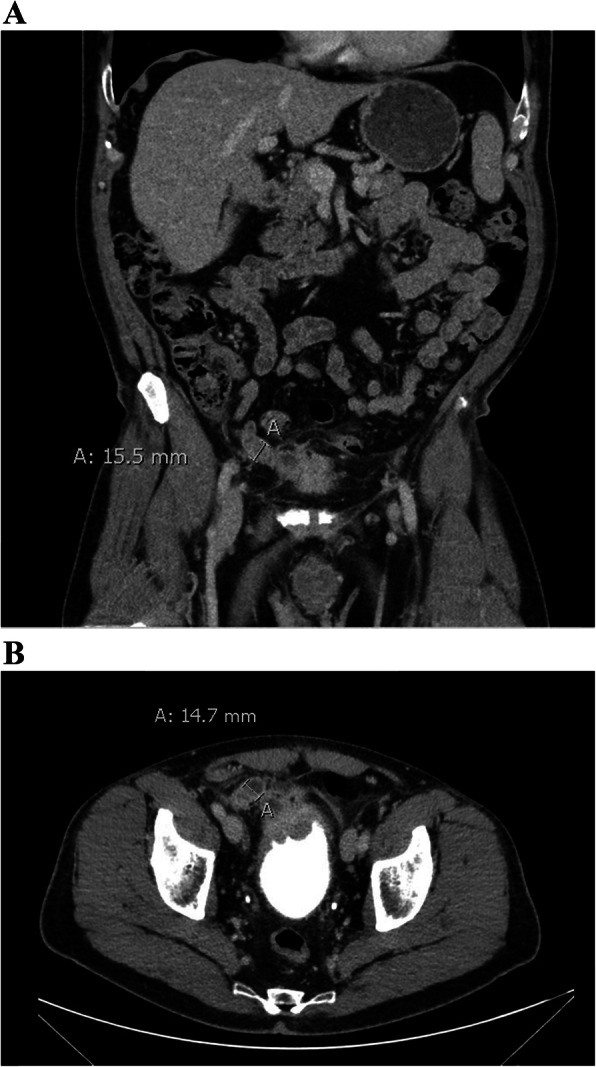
CT with contrast of abdomen and pelvis. a. CT with contrast of abdomen and pelvis (coronal plane). An estimated appendiceal luminal dilation of 15.5 mm (left) with fistula to the bladder is displayed. b. CT with contrast of abdomen and pelvis (axial plane). An estimated appendiceal luminal dilation of 14.7 mm (left), with fistula formation to the bladder is displayed
